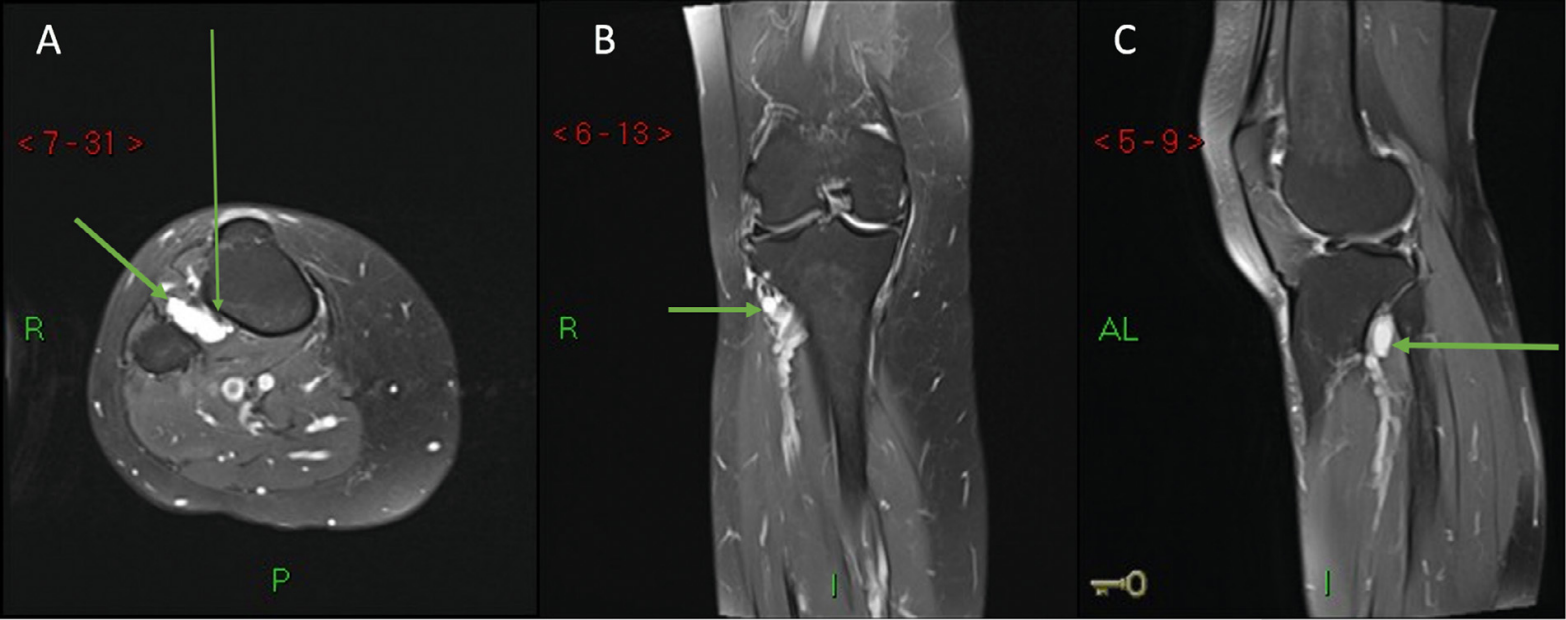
(A, B, C): Right knee MRI in axial (A), coronal (B) and sagittal (C) Proton Density Fat Suppressed showing a juxta-articular ganglion cyst (thick arrow) located in the interosseus space immediately inferior and in communication with proximal tibiofibular joint space. The cyst is causing compression of the deep peroneal nerve (thin arrow). R is right; AL is antero-lateral; P is posterior.
